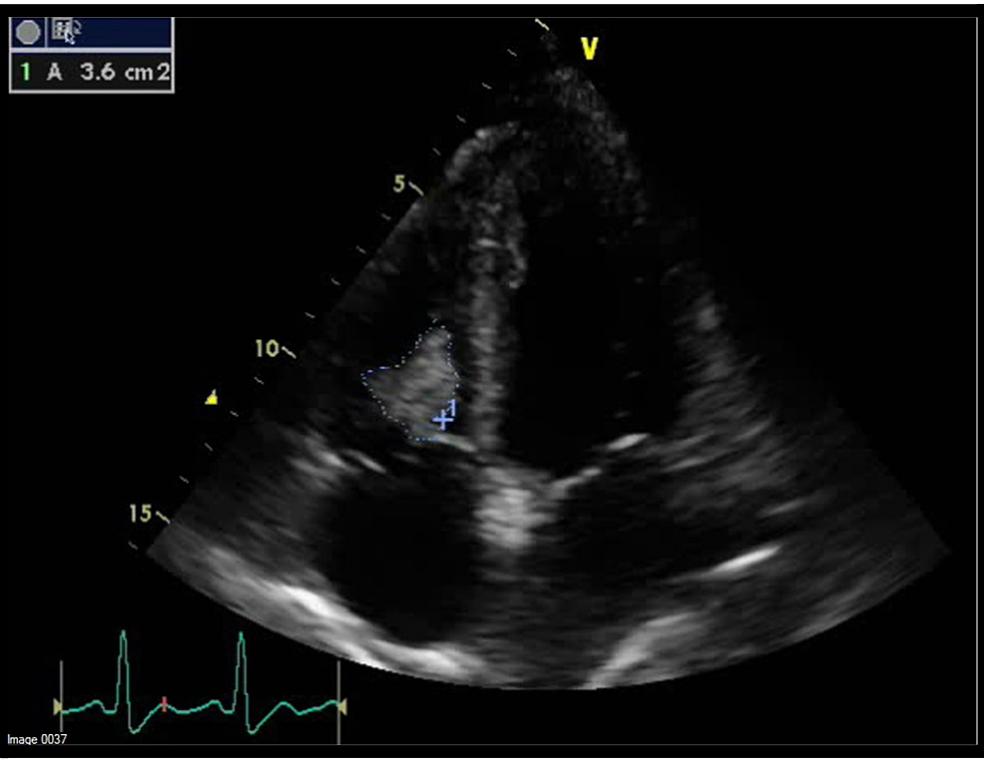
Transthoracic echocardiogram imaging with the echogenic mass in the right ventricle obstructing the tricuspid valve
